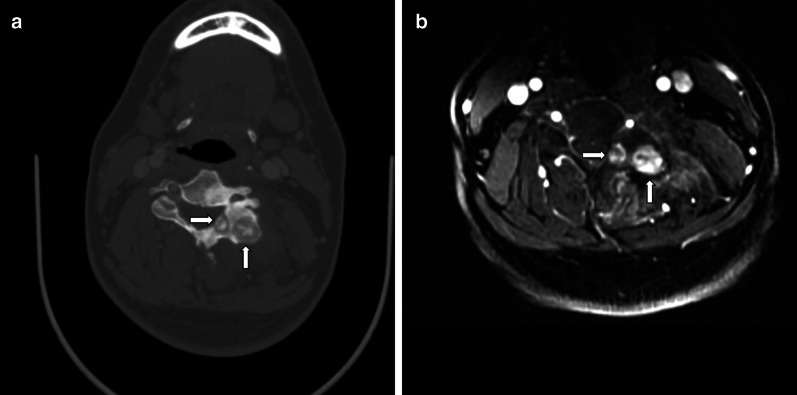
A 31-year-old man with multifocal lesions. a Shows representative images of with a lesion on the left accessory of C4. CT shows two obvious niduses in the accessory. Contrast enhancement on MRI can show the lesions well, with both the nidus and surrounding edema exhibiting intense enhancement (b)
